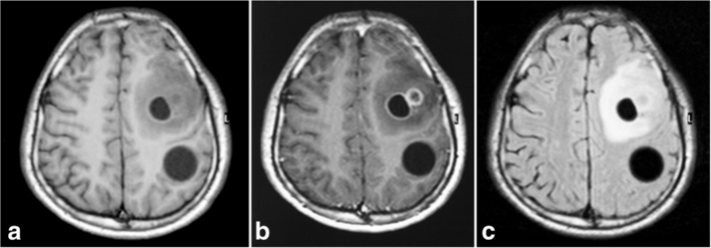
Brain MRI. Pre (a), Post contrast (b), T1 Weighted images and FLAIR (c) sequences showing multiple intraparenchymal brain cysts associated with perilesional edema.
